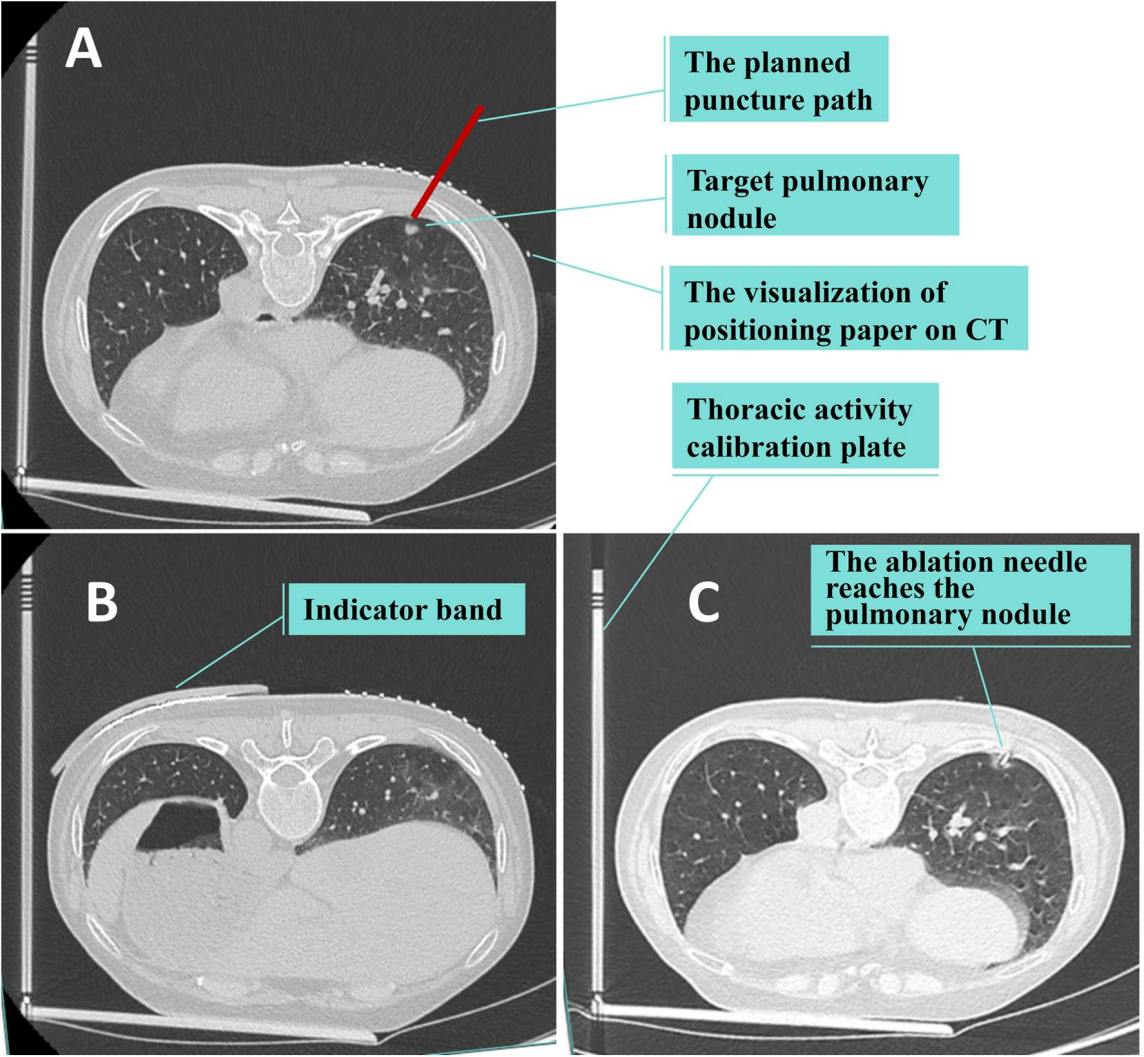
Ct scan. (A,B) The first CT scan is conducted to determine the puncture point, puncture level, and the scale of the thoracic activity calibration plate indicated by the attached indicator band on the chest skin. (C) The second CT scan is conducted again to determine whether the puncture needle has reached the predetermined position.
